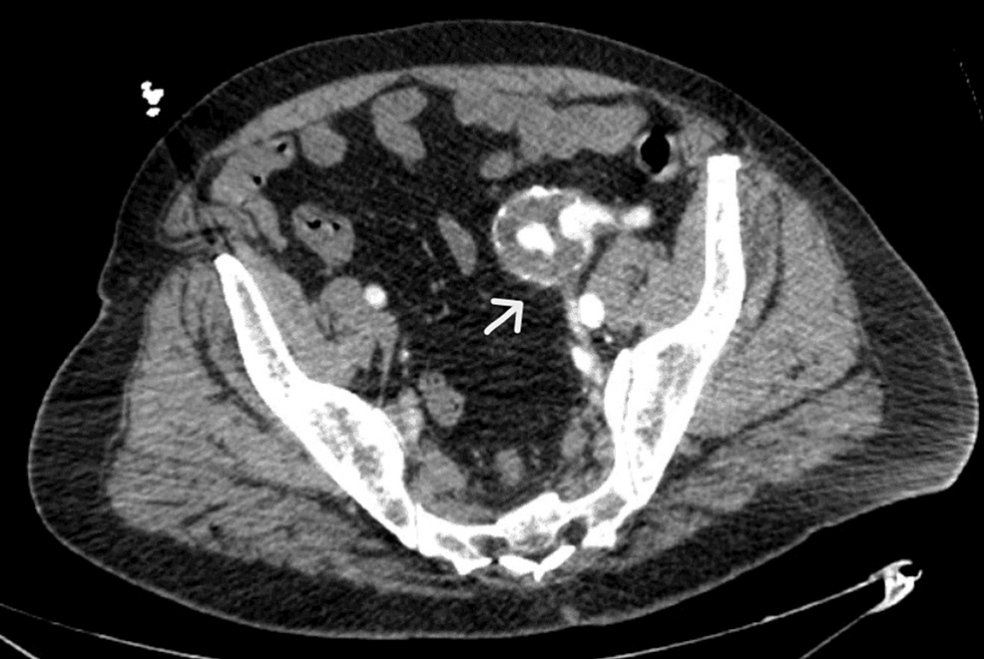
CT with IV contrast demonstrating an iliac artery aneurysm with an AV fistula (arrow)
